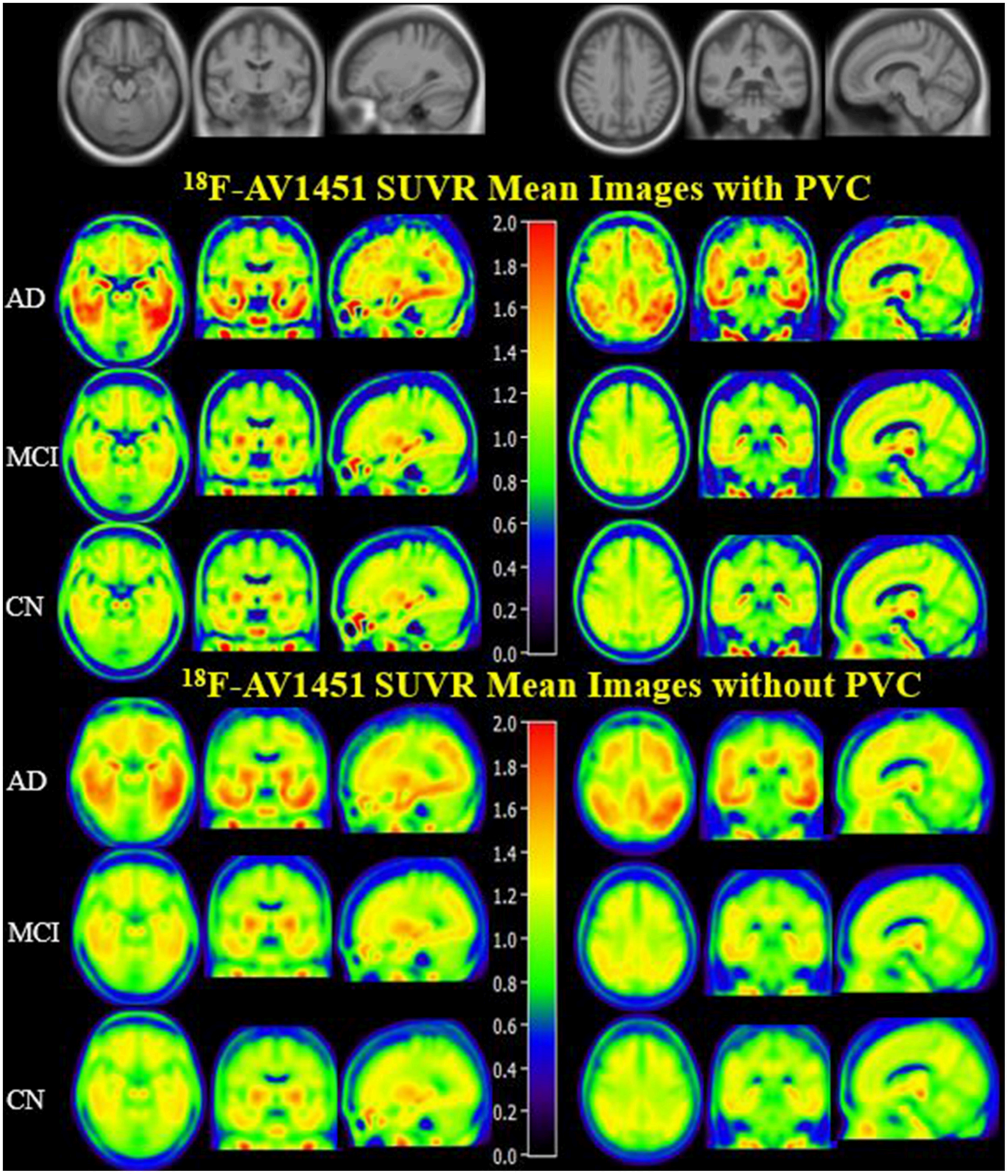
^18^F-AV1451 SUVR Mean Images with PVC and non-PVC. Mean images generated by computing the mean of images from AD, MCI, and CN participants separately. The upper images have been corrected with PVC, whereas the lower images without PVC.
